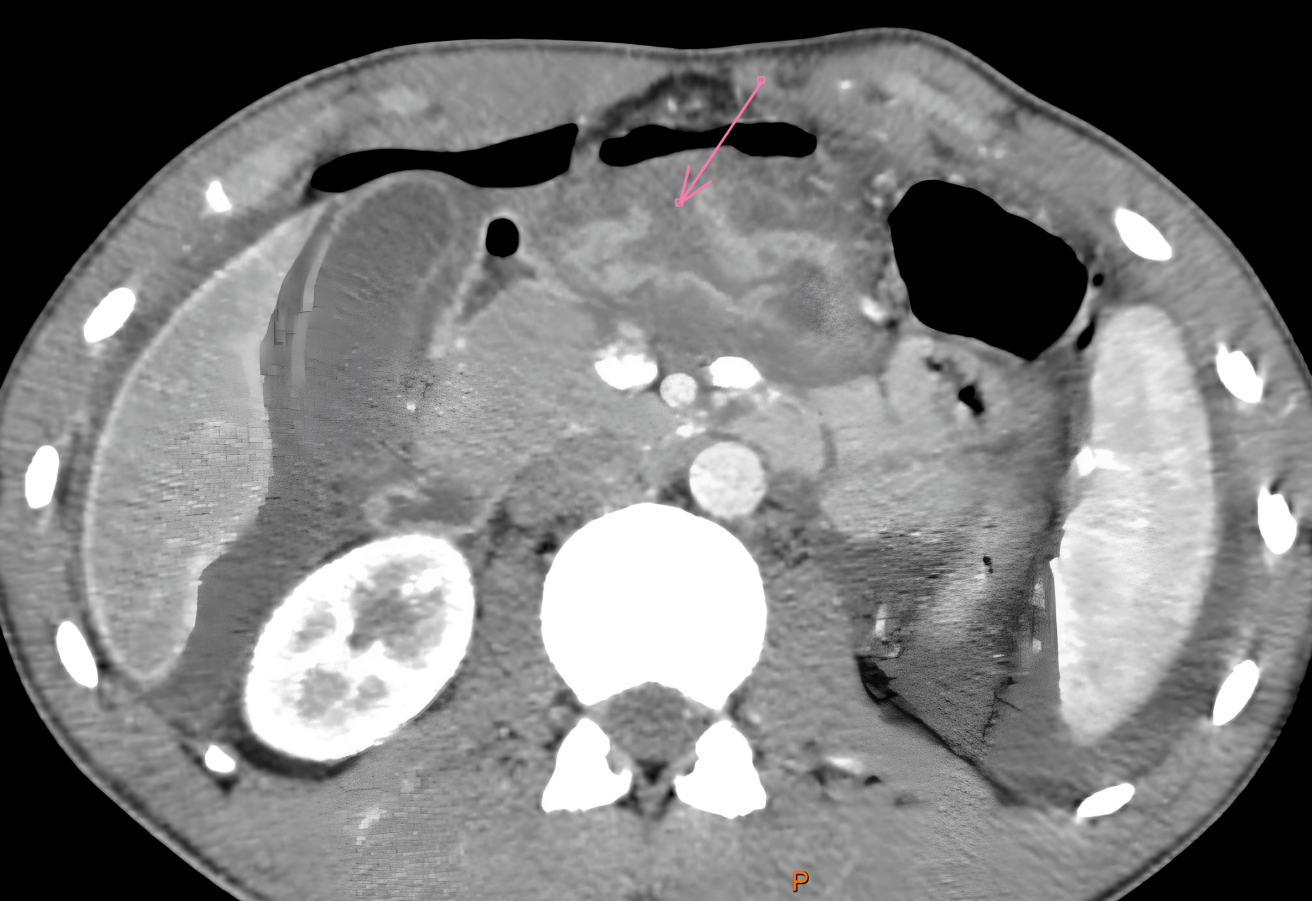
Axial computed tomography slice revealing an anterior gastric perforation
